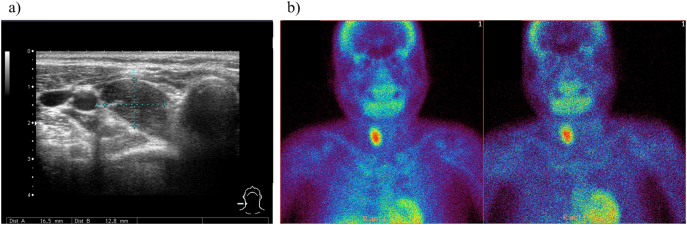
a) In case 2, ultrasonography revealed an 8 mm-solid mass indicated as D1 localized in the left inferior thyroid gland. b) ^99m^Tc-MIBI scintigraphy identified a hyperfunctioning left inferior parathyroid gland in case 2.
